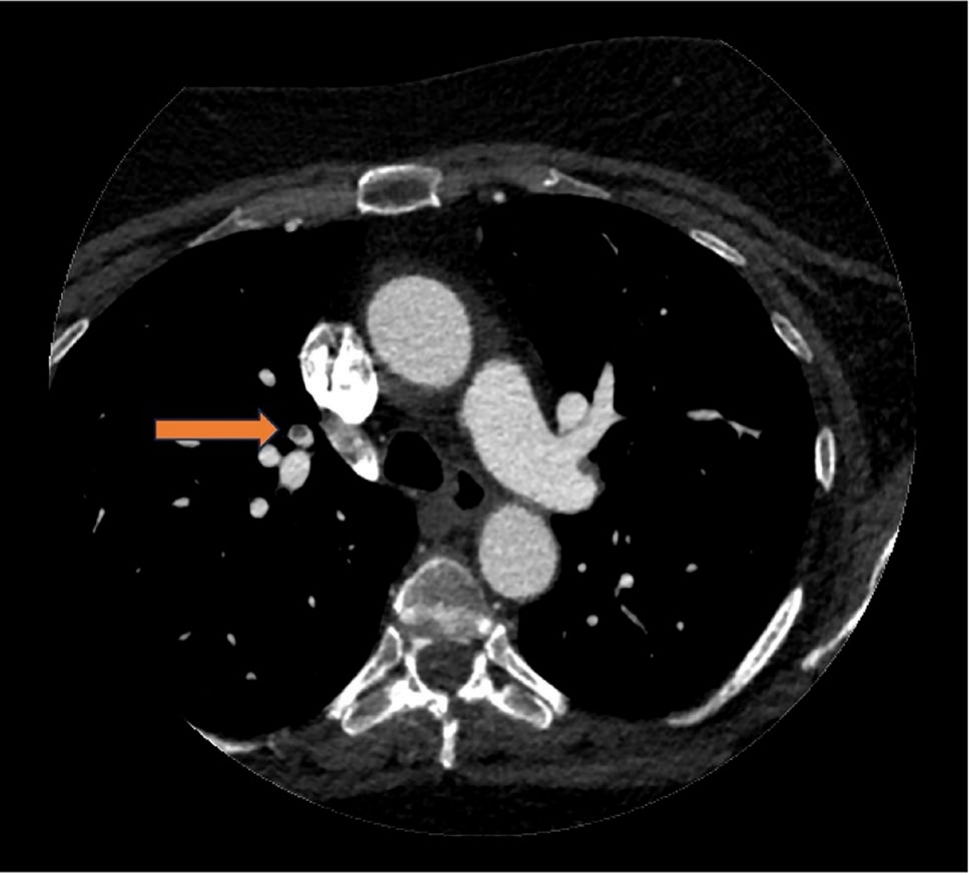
Right upper lobe segmental pulmonary embolism (PE) identified in a coronary artery CTA from an asymptomatic 60-year-old female with cardiovascular risk factors. Initially unreported in the original radiology report, this PE was later detected by the AI algorithm
